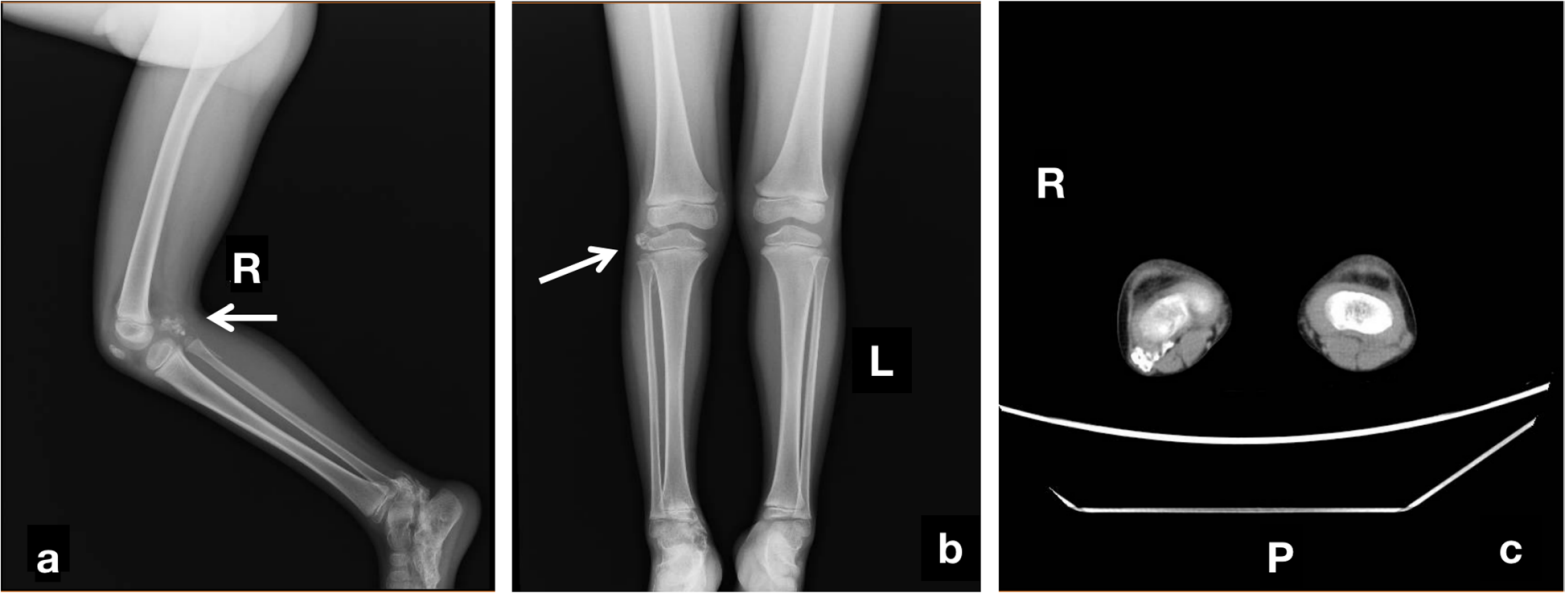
Radiographs of the right keen. Lateral and anteroposterior radiograph of the legs shows ossification (arrows) in the soft connective tissues from the dermis down through the muscle to the proximal tibi (a, b). Axial CT shows ossification has fused with the tibia (c)
